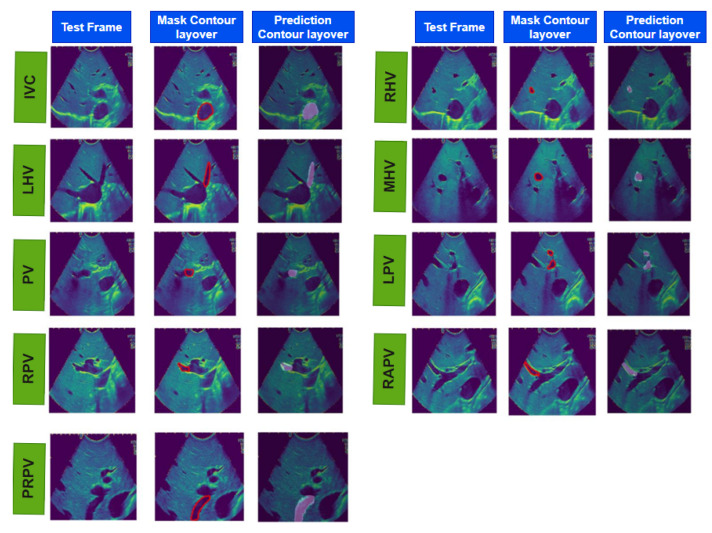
The results of real-time liver vessel segmentation on intraoperative ultrasound video frames using our proposed 2D-weighted enriched U-Net model. The figure showcases test images, mask contour layover images, and the corresponding predicted contour layover images generated by the model for multiple intraoperative ultrasound video frames.
